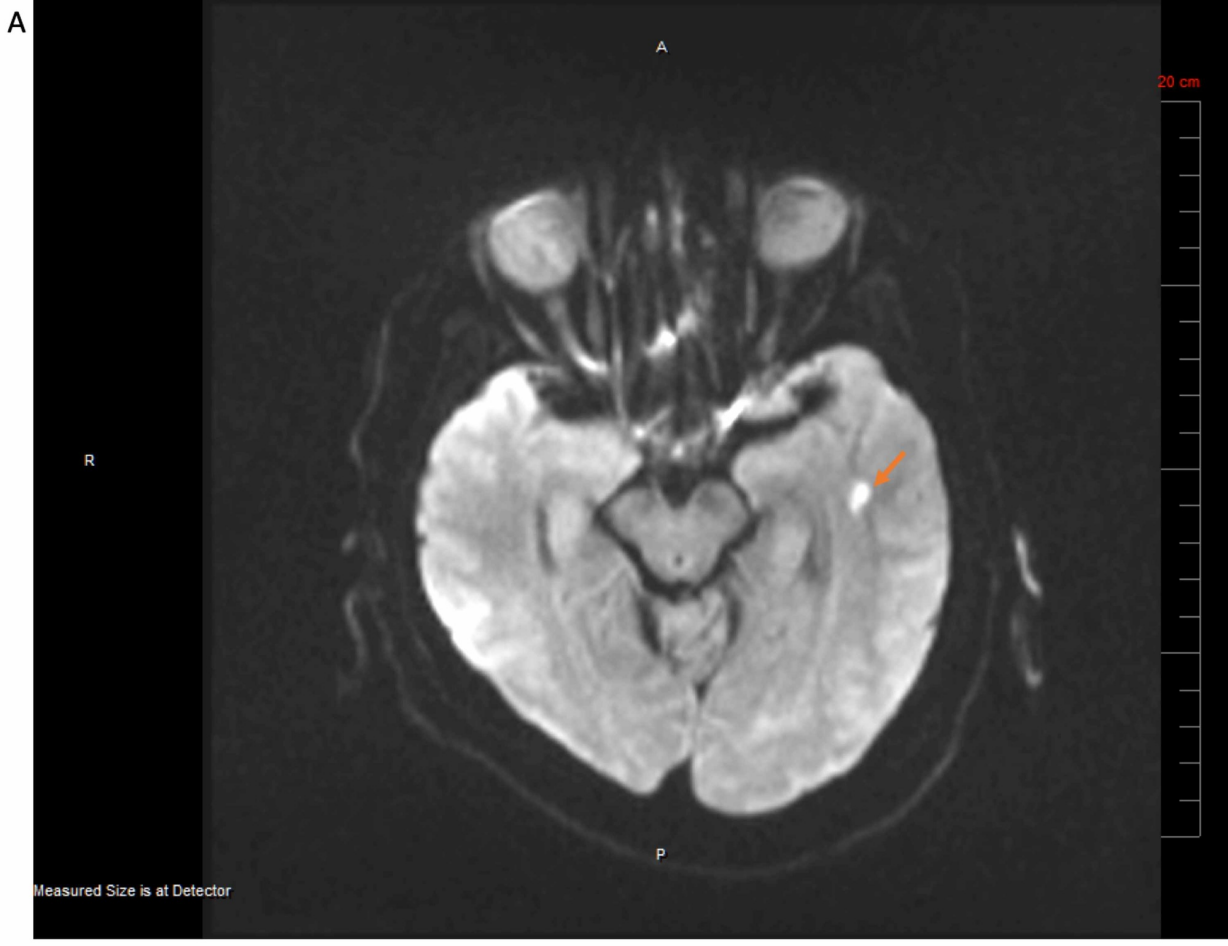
Acute Non-hemorrhagic Infarct in a Patient with Mild COVID-19 There is a small acute non-hemorrhagic infarct in the left anterior temporal lobe white matter on MRI.
